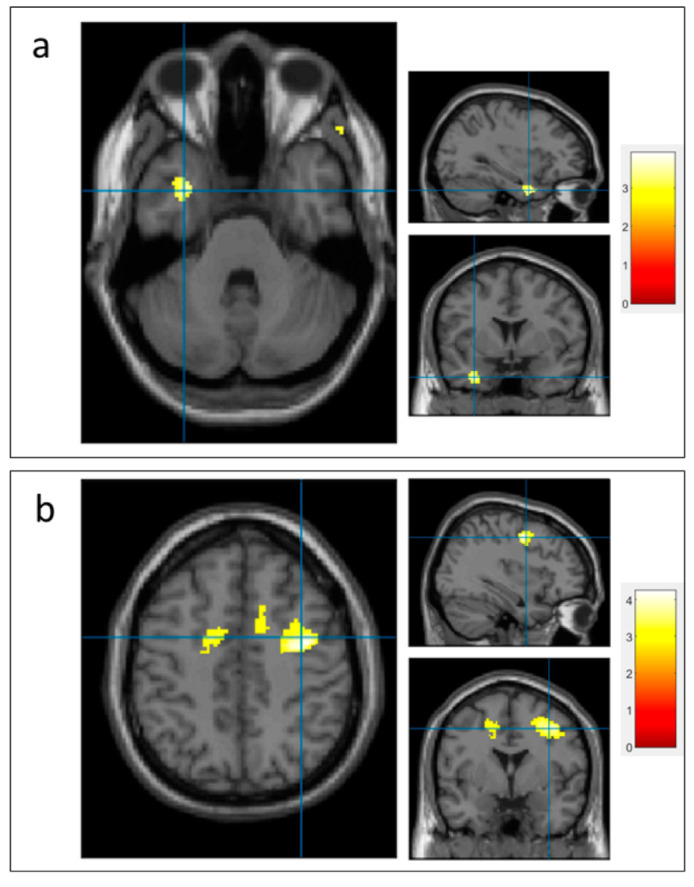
Functional MRI showing brain area activated during the acquaintance face recognition task, without device assistance (a) and with device assistance (b).
